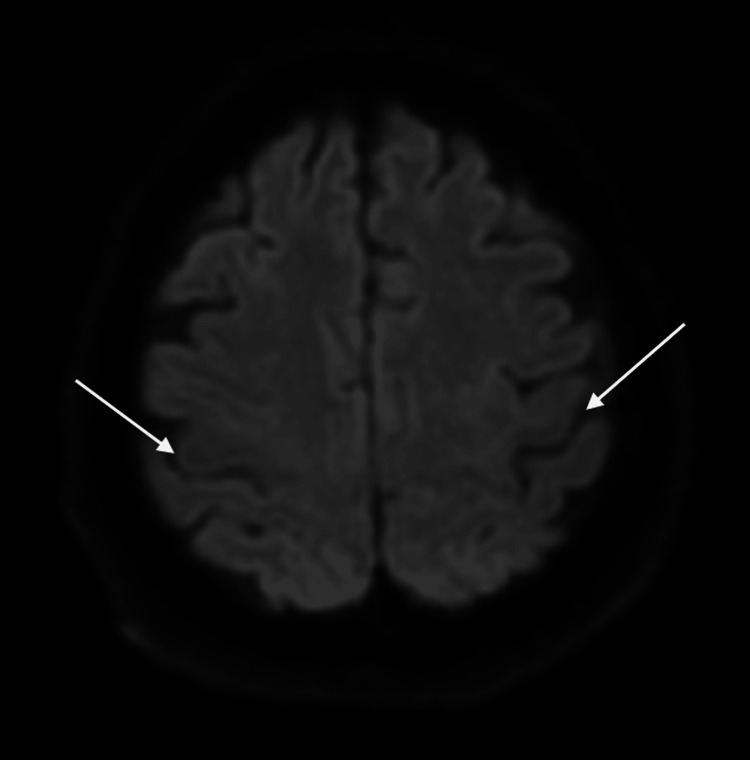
The MRI of the brain shows an axial view and diffusion-weighted imaging (DWI). Arrows pointing at the central sulci; there is no diffusion restriction in the precentral gyri anterior to the central sulci or any other area.
